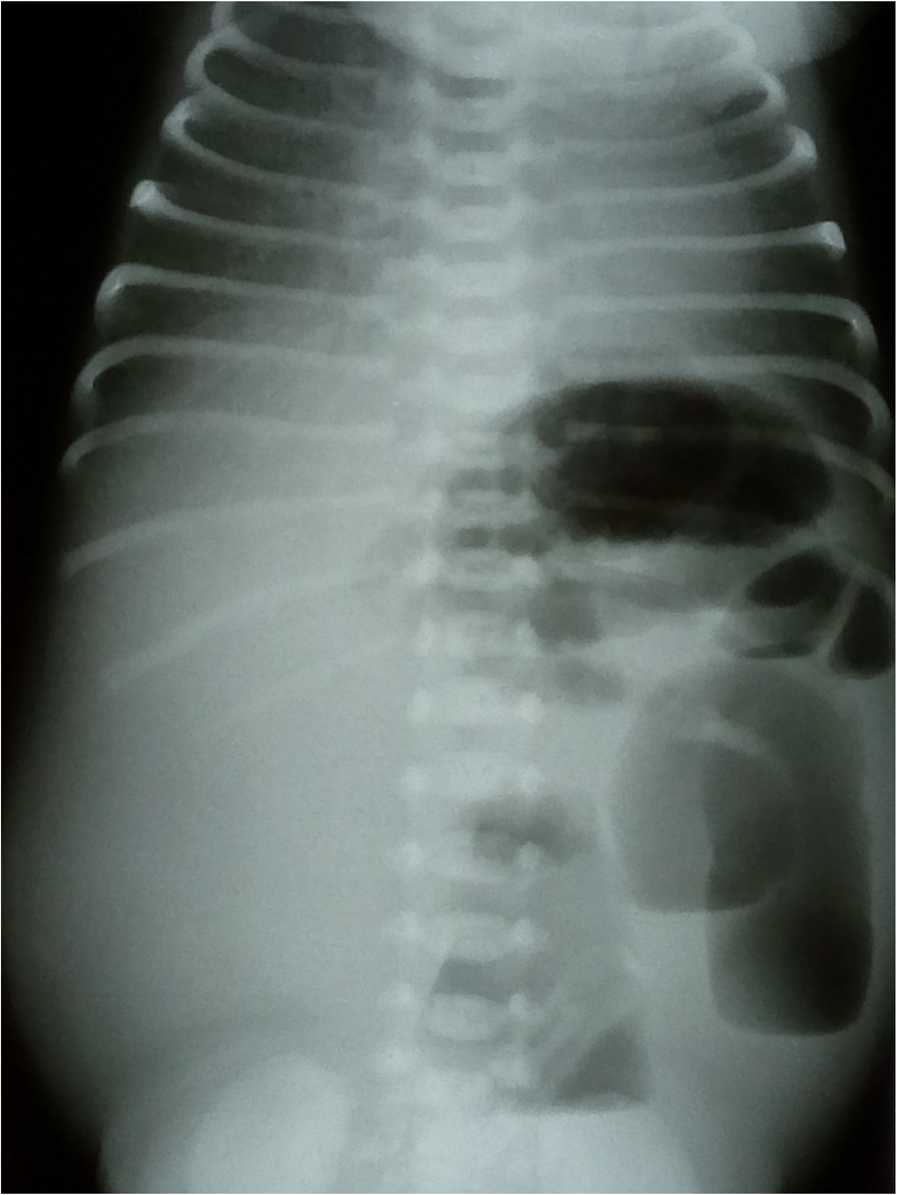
Simple abdominal radiography. Imaging study on the first life day shows small bowel ileus
